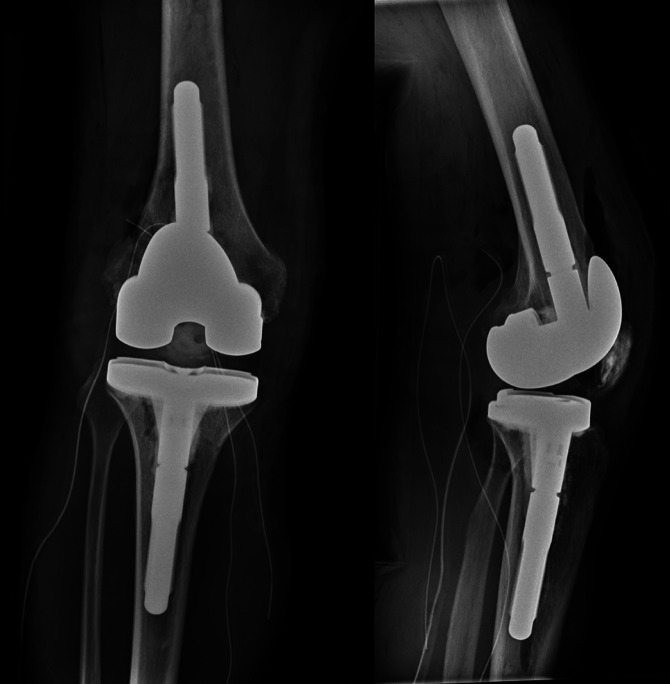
AP (left) and lateral (right) postoperative radiographs showing right knee revision total knee arthroplasty.
